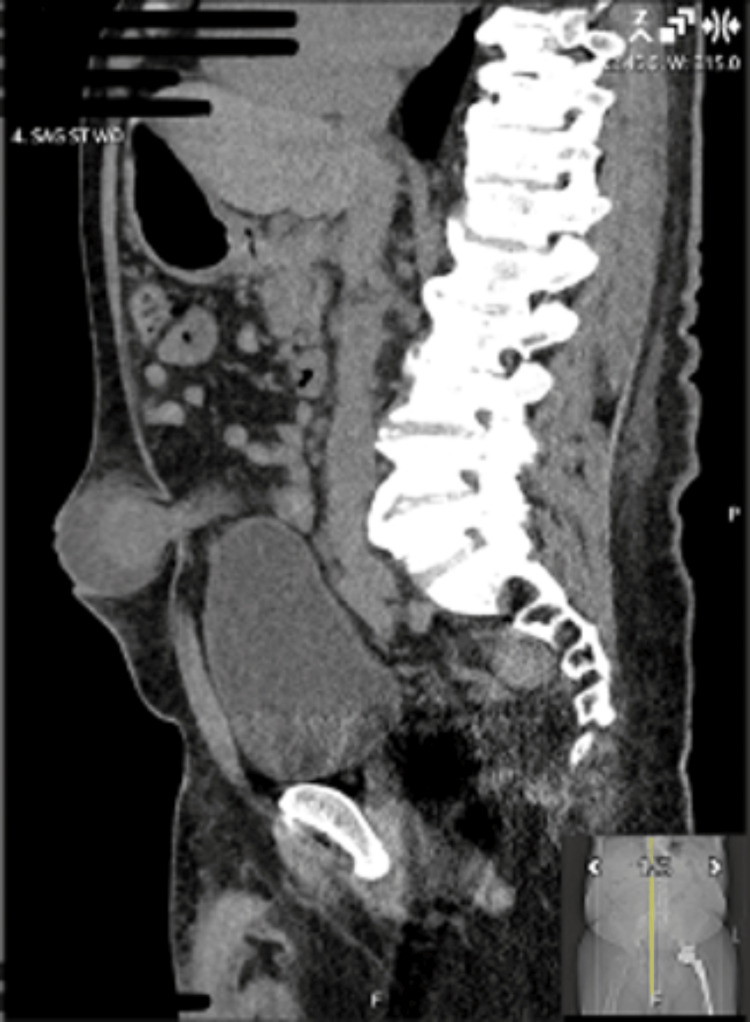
Sagittal computed tomography image demonstrating a protruding umbilical hernia sac consistent with incarceration
